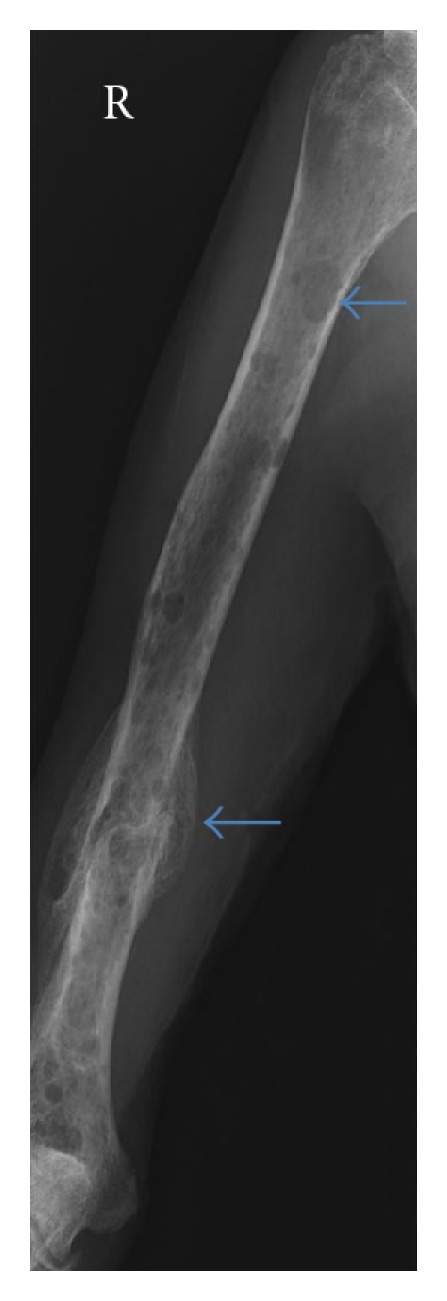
A-P radiograph right humerus: diffuse lytic lesions of the right humerus (arrowed) with old pathological fracture distal diaphysis (arrow).
